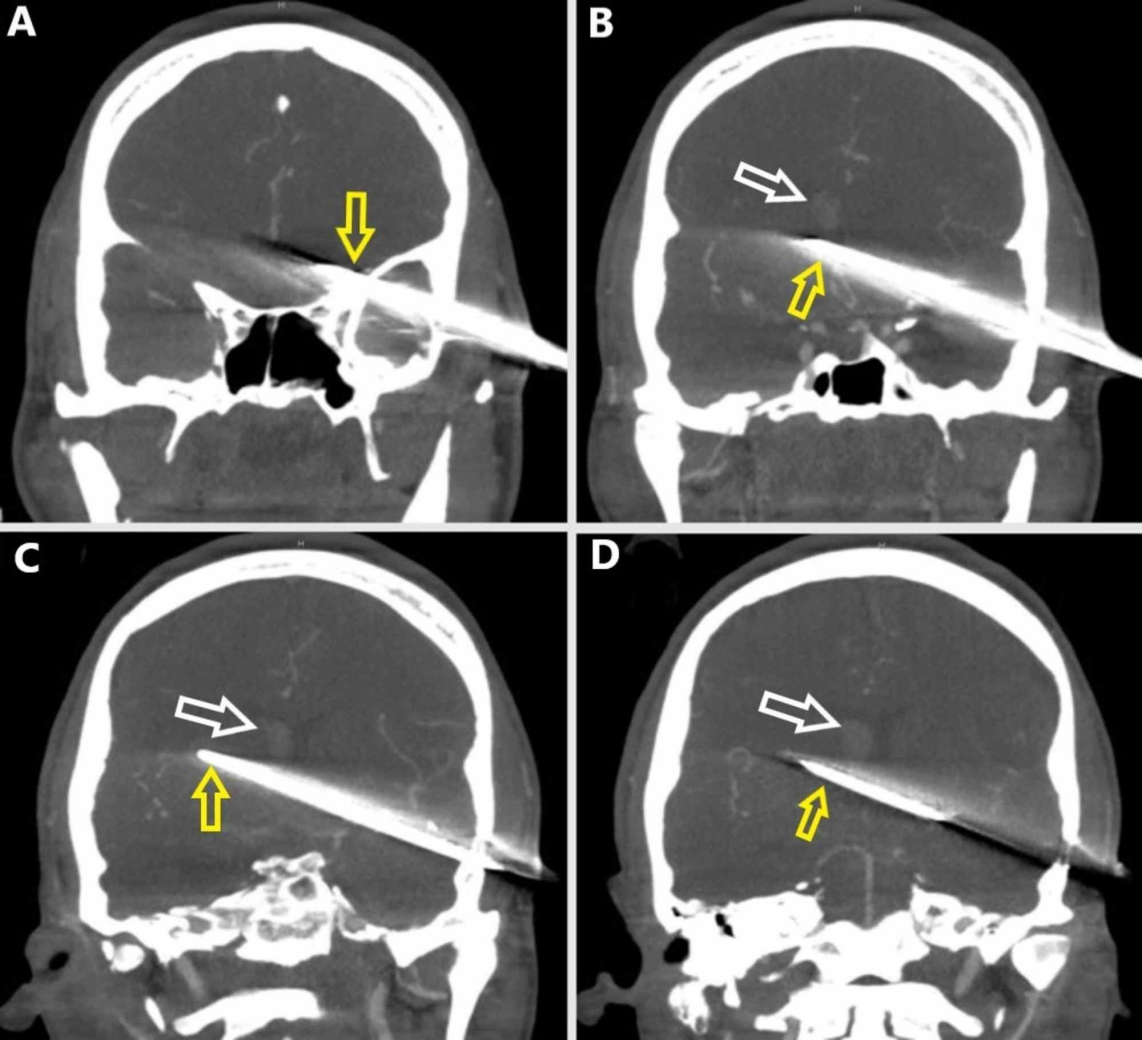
Coronal plane preoperative CT angiography in the vascular window setting progressing from anterior (A) to posterior (D). (A) The knife (yellow arrow) penetrates the left temporal bone. (B) The knife (yellow arrow) passes midline, and a right lateral intraventricular hemorrhage is evident (white arrow). (C) The knife is seen along the full extent of its intracranial route (yellow arrow). The right lateral intraventricular hemorrhage is evident (white arrow). (D) Posterior limit of the intracranial knife (yellow arrow). The right lateral intraventricular hemorrhage is evident (white arrow).
